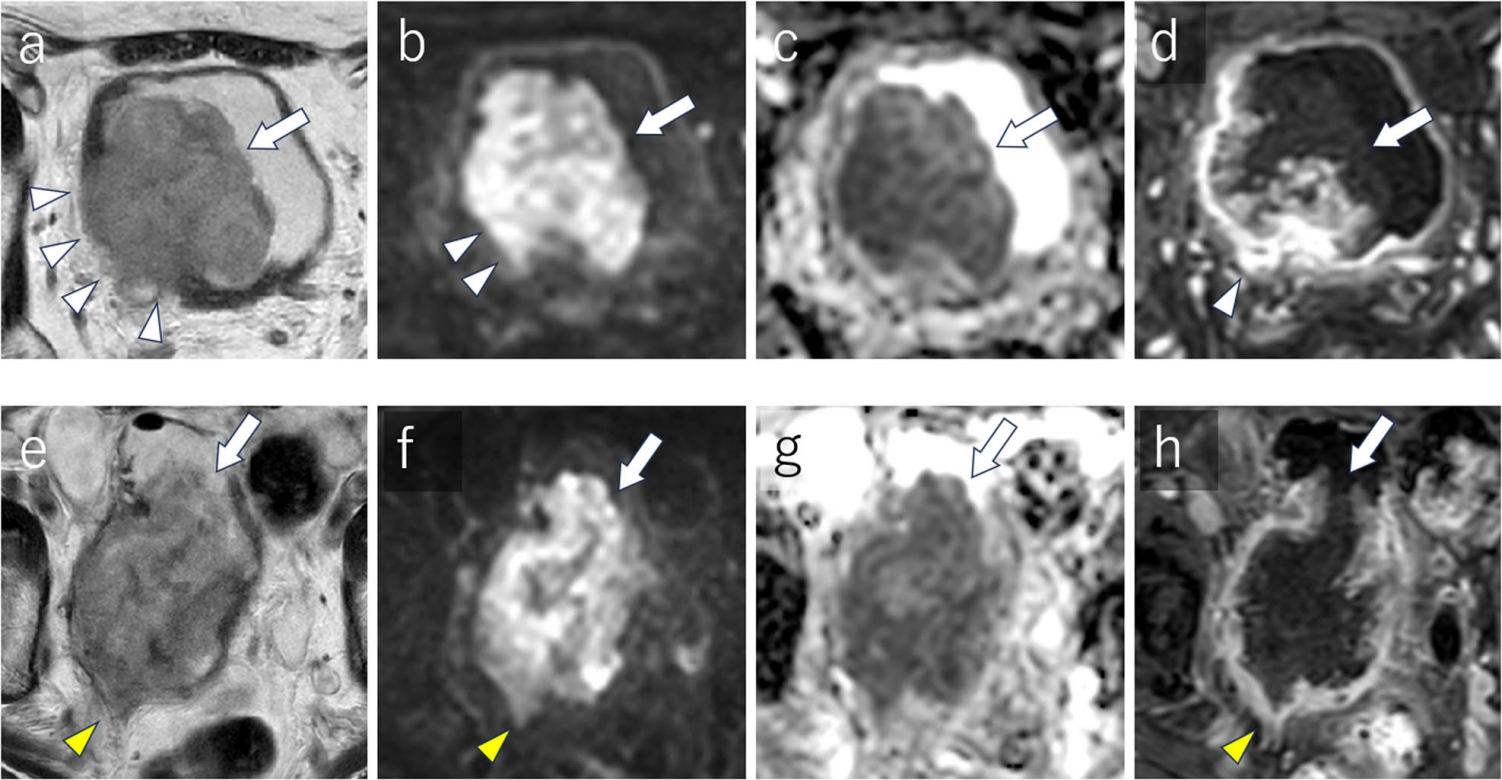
NAC non-responder: a 71-year-old man with a 65-mm urothelial carcinoma with squamous differentiation the right posterior bladder wall Pre-NAC MRI reveals a lobulated tumor with partial disruption in the muscle layer on T2WI (a) [T2WI VI-RADS score: 4], high-signal intensity on DW-MRI indicating infiltration into the muscle propia (b) [DW VI-RADS score: 4], heterogeneous low signal intensity on the ADC map with a mean ADC value of 0.994 × 10^−3^ mm^2^/s (c), and DCE-MRI shows heterogeneous enhancement and muscle layer disruption (d) [DCE VI-RADS score: 4]. After one course of gemcitabine and cisplatin, and two courses of gemcitabine and docetaxel due to renal dysfunction, post-NAC MRI shows tumor expansion and invasion into the perivesical fat on T2WI (e) [T2WI VI-RADS: 5] and DW-MRI (f) [DW VI-RADS: 5], with a corresponding low signal intensity on the ADC map (a mean ADC value: 0.906 × 10^−3^ mm^2^/s) (g). DCE-MRI confirms perivesical infiltration (h) [DCE VI-RADS: 5]. The tumor is indicate white arrows, muscle infiltration by white arrowheads, and perivesical fat infiltration by yellow arrowheads. ADC, apparent diffusion coefficient; DCE, dynamic contrast-enhanced imaging; DW, diffusion-weighted neoadjuvant chemotherapy; T2WI, T2-weighted imaging; VI-RADS, vesical imaging-reporting and data system
